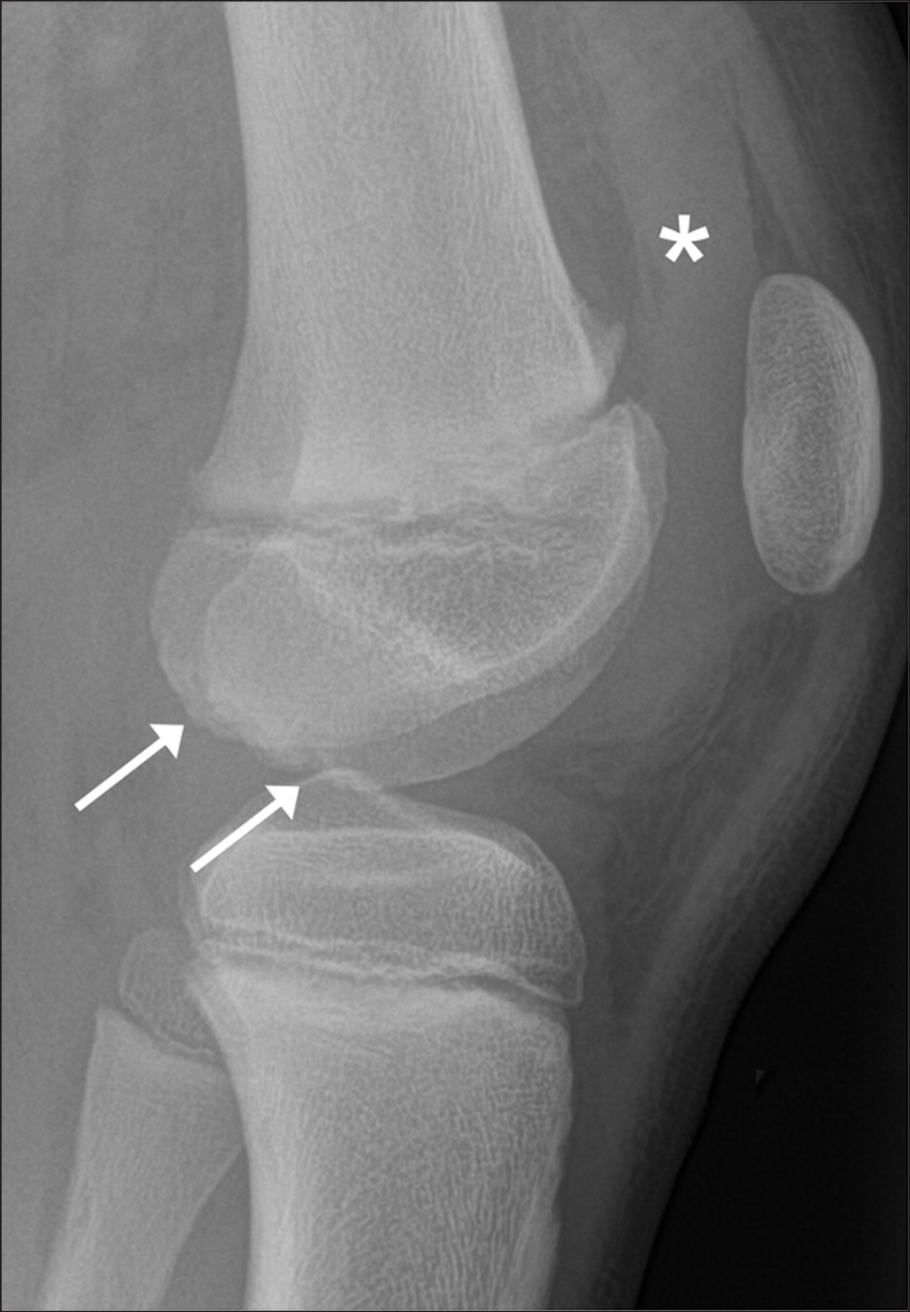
MRI of the right knee – proton density (PD) and fat suppressed proton density (FS-PD) – were obtained to evaluate for radiographically occult fractures and meniscal or ligamentous injury. MRI evidenced a Salter-Harris type III fracture (*Figure 2, PD-sequence, short arrow*) with widening of the growth plate (*Figure 2, PD-sequence, long arrow*).
